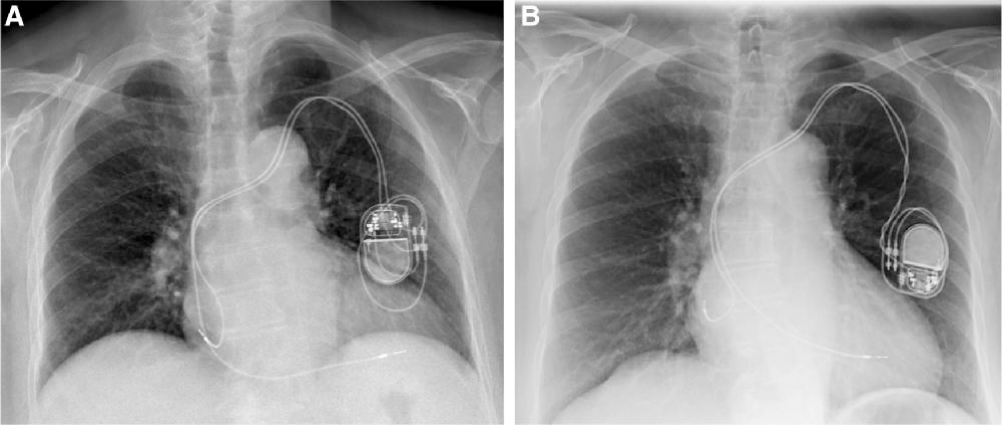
Case report 2. (*A*) Post-revision chest X-ray. (*B*) Chest X-ray after lead repositioning.
